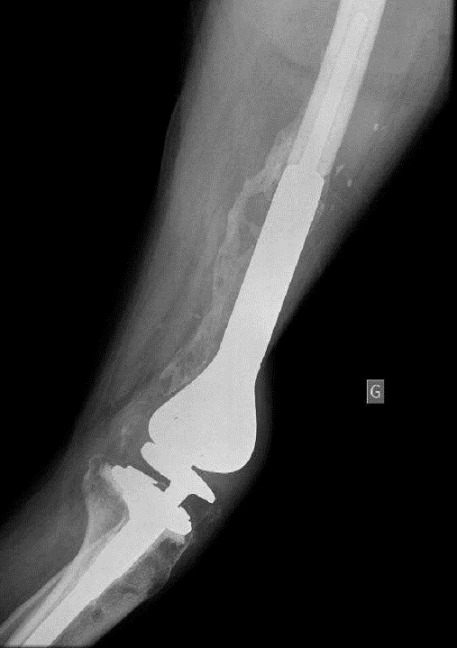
Lateral radiograph of the left knee showing pseudo-synovial membrane ossification around the knee megaprosthesis
